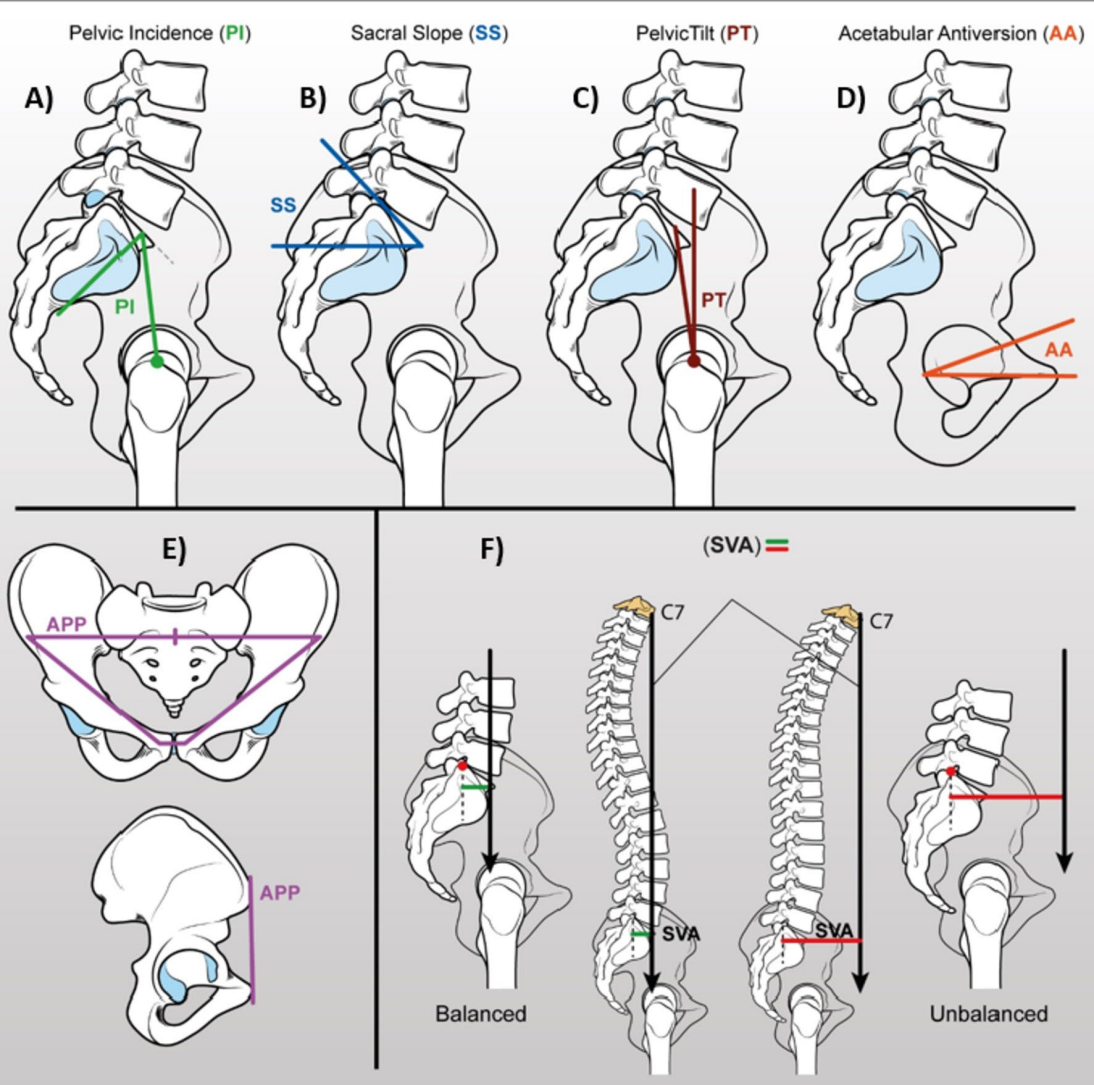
A Pelvic Incidence (PI): is an angle formed by a line perpendicular to the S1 endplate and a second line from the midpoint of S1 to the center of the bicoxofemoral axis. The PI value indicates the pelvis' ability to compensate the sagittal imbalance of the spine by rotation around the bicoxofemoral axis: the higher the PI, the greater the possibility of pelvic retroversion. It is equal to the sum of SS and PT; this relationship justifies the inverse relationship between SS and PT. B Sacral Slope (SS): is an angle formed by the S1 endplate and a horizontal line. High SS values imply a horizontal orientation of the sacrum (anteverted pelvis), while negative values make standing impossible. C Pelvic Tilt (PT): is an angle formed by a line from the midpoint of S1 endplate to the center of the distance between two femoral heads and a vertical line. It indicates the spatial orientation of the pelvis, which varies depending on the position assumed by the patient during standing and walking. It is complementary to SS: considering the rotation of the pelvis around the bicoxofemoral axis, PT increases when the pelvis rotates backward (retroversion) and decreases when the pelvis rotates forward (anteversion). D Acetbular Anteversion (AA): is an angle formed by a line through the long axis of the acetabulum and a horizontal line measured on sagittal radiographs. E Anterior Pelvic Plane (APP): a plane formed by the anterior–superior iliac spines (ASIS) and the pubic symphysis. In neutral spinal balance this plane corresponds to the Functional Pelvic Plane (FPP), a vertical plane through the pubic symphysis, the ASIS and perpendicular to the ground. F Sagittal Vertical Axis (SVA): Horizontal distance between a plumb line starting from the body of C7 and running perpendicular to the ground and the posterosuperior angle of S1. This parameter describes the overall sagittal balance of the thoracolumbar spine and it is considered physiological within 5 cm. Values between 5 and 15 cm outline a partial compensation, while decompensated alignment results above 15 cm
